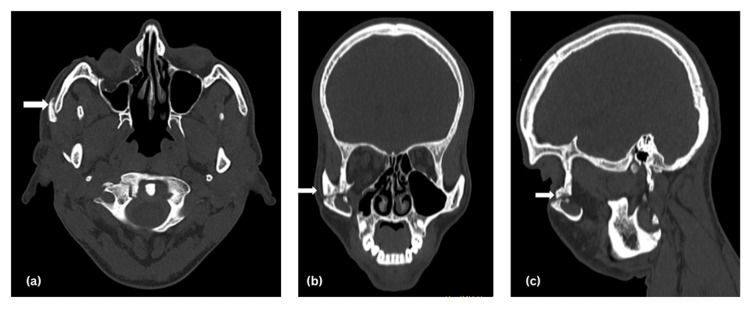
(a) Axial, (b) coronal, and (c) sagittal views of head CT showing the malunited zygomaticomaxillary complex fracture.
